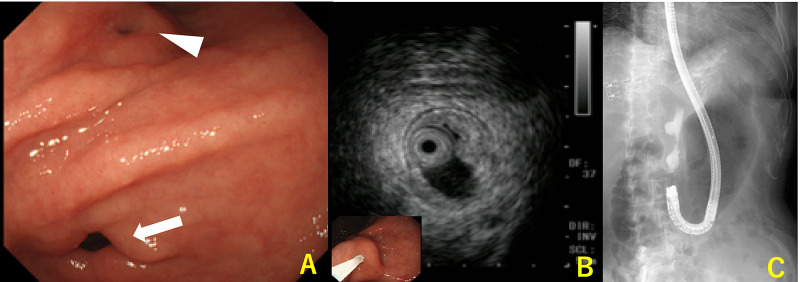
Pre-operative images Upper gastrointestinal endoscopy shows a small orifice along the lesser curvature of the lower body of the stomach; the pyloric ring (arrow) and orifice of the duplication cyst (arrowhead) are seen (A). Endoscopic ultrasound shows a tubular lesion lined by a mucosal layer with the surrounding muscularis propria visible in the root of the gastric cyst, suggestive of a duplication cyst (B). Contrast fistulography shows a tubular lesion, 7 cm in length, with a stricture in the middle portion of the lesion (C).
